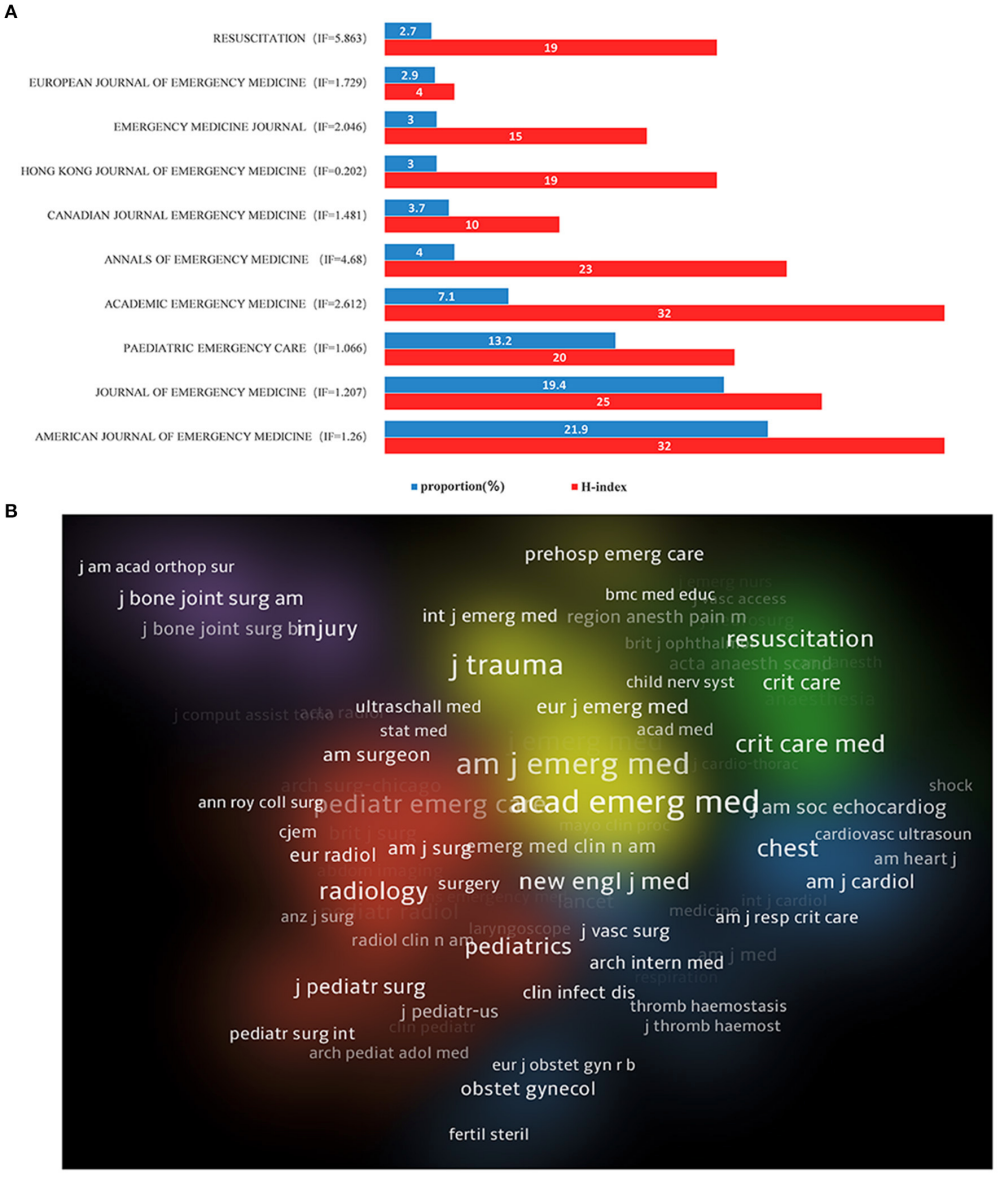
The distribution of journals engaged in research on the use of ultrasound in emergency medicine. (A) Top 10 journals by number of publications, blue bar representing the proportion and red bar representing H-index (B). The network of institutions produced in VOSviewer, the size of circles reveals the H-index.
